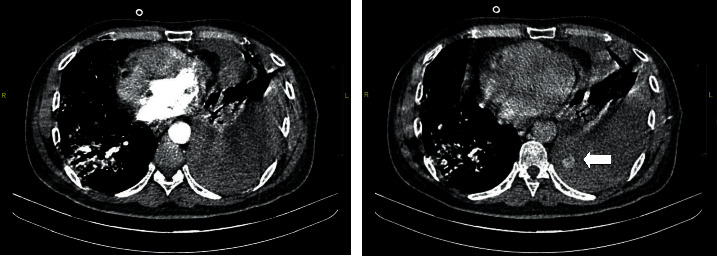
CT angiography of the thorax demonstrating a streak of contrast extravasation arising from 4^th^ intercostal space on portovenous phase with further pooling of contrast into pleural cavity at a 3-minute delayed phase (shown by the arrow).
